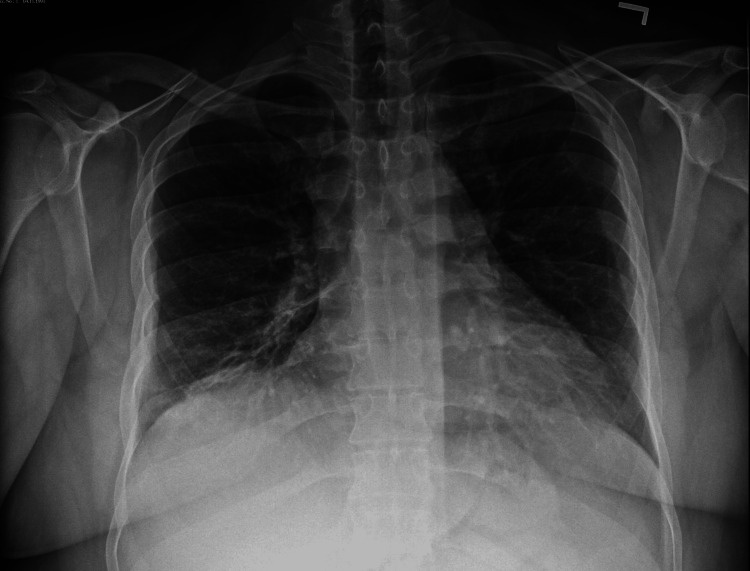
AP chest X-ray showing improved right lower lobe consolidation at a follow-up visit nine days later.
